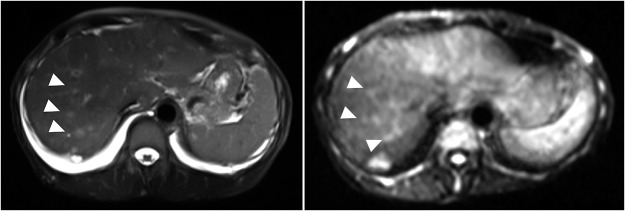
Axial T2-weighted and diffusion-weighted MRI examination of the liver. Multiple hyperintense areas are diffusely distributed throughout the liver parenchyma, as indicated by white triangles. These findings are consistent with occult, infiltrative liver metastases from breast cancer, lacking discrete nodular formation.
